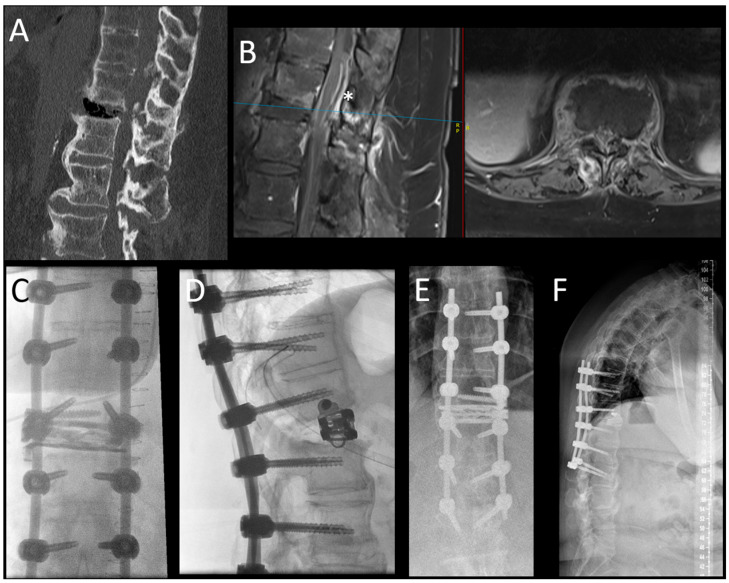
This picture demonstrates the case of a 59-year-old woman who presented with a B3-type injury of the T12-L1 segment in the setting of an ankylosed spine (Bechterew’s disease). In the upper row, the sagittal view of the CT scan (A) demonstrates the extension injury of the segment with massive air collection in the ruptured disc space and the typical findings of a Bechterew’s disease with ossification of ALL and the posterior ligaments. In the sagittal and axial MRI scan (separated by a red line) (B), an epidural hematoma with compression of the conus (*) can be appreciated. The blue line indicates the height of the axial imaging on the sagittal imaging. The intraoperative (C,D) and postoperative (E,F) images are shown in the lower row. Here, the posterior stabilization was performed in an open fashion because a spinal canal decompression was needed; the construct was chosen as long-construct fusion (3 levels above and below the injury) due to the ankylosed spine. In this case, the LLIF procedure was performed in a separate anesthesia. With the ELSA expandable spacer, the gapping defect at the level of the disc T12-L1 was filled, and the spacer was secured with the additional screw to avoid dislocation. The additional anterior column support helped to keep the lordosis of this segment, gained by the injury, in a globally kyphotic spine.
